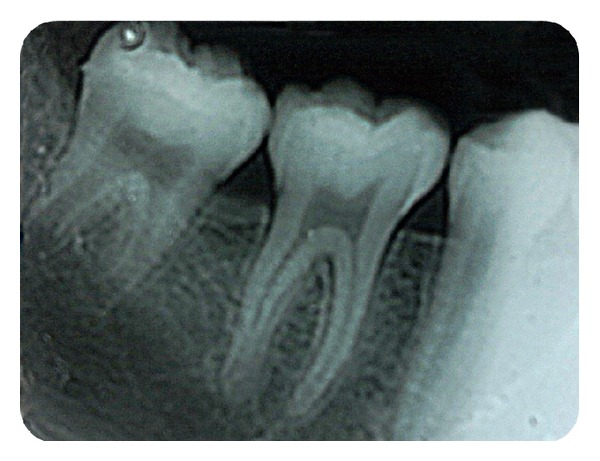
Posttreatment intraoral periapical radiograph showing erupted permanent mandibular second molar erupted.
